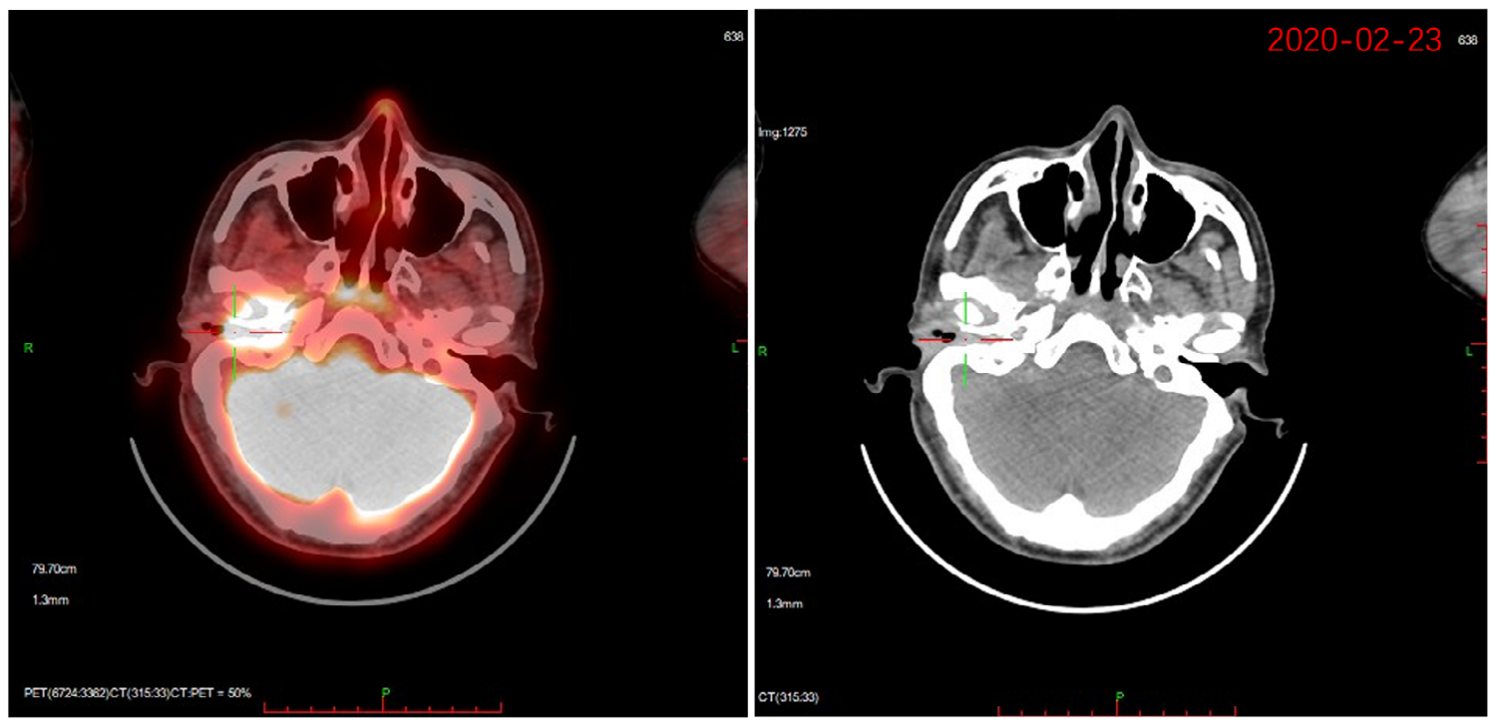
18F‐FDG PET‐CT reveals a slight increase in soft tissue density in the right ear region with elevated metabolism, with an SUVmax of approximately 10.8. ^18^F‐FDG, ^18^F‐fluorodeoxyglucose; PET‐CT, positron emission tomography‐computed tomography.
